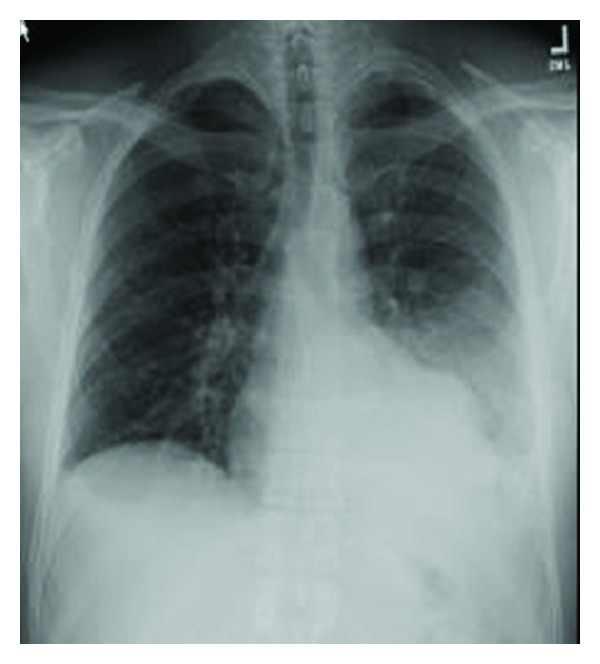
Chest X-ray on day 1 of patient 1.
